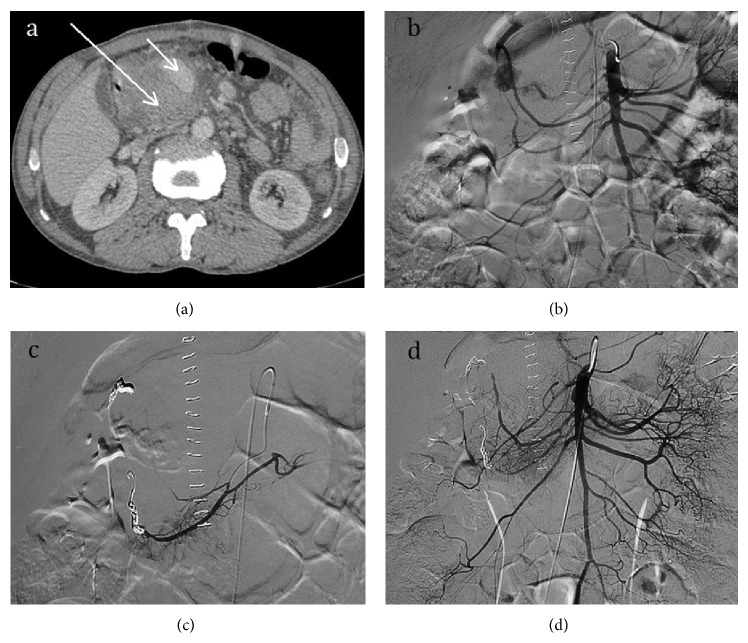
(a) Contrast-enhanced axial CT image shows a giant pseudoaneurysm of 8.3 × 7.5 cm in size originating from the gastroduodenal artery (long arrow). The intravenous contrast showed filling of the mass, certifying its vascular origin (short arrow). (b) Aortography shows the blood circulation of the aneurysm in continuity with the gastroduodenal artery. (c) Angiogram after selective coil-embolization of the gastroduodenal artery through the celiac trunk and the inferior pancreaticoduodenal artery, through the superior mesenteric artery. (d) Angiographic control confirmed the complete exclusion of the pseudoaneurysm.
